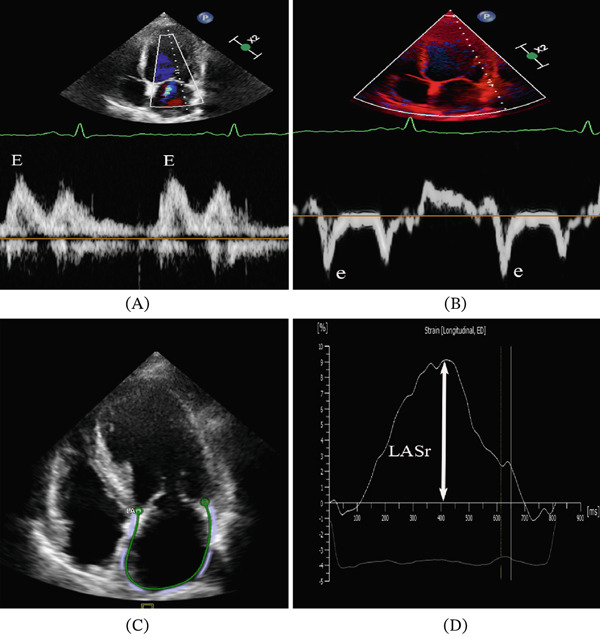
The assessment of left atrial stiffness by echocardiography. (A) Measurement of mitral inflow E‐wave velocity. (B) Evaluation of lateral mitral annular e′ velocity. (C) Analysis of left atrial strain. (D) Left atrial reservoir strain as shown on the left atrial strain curve.
